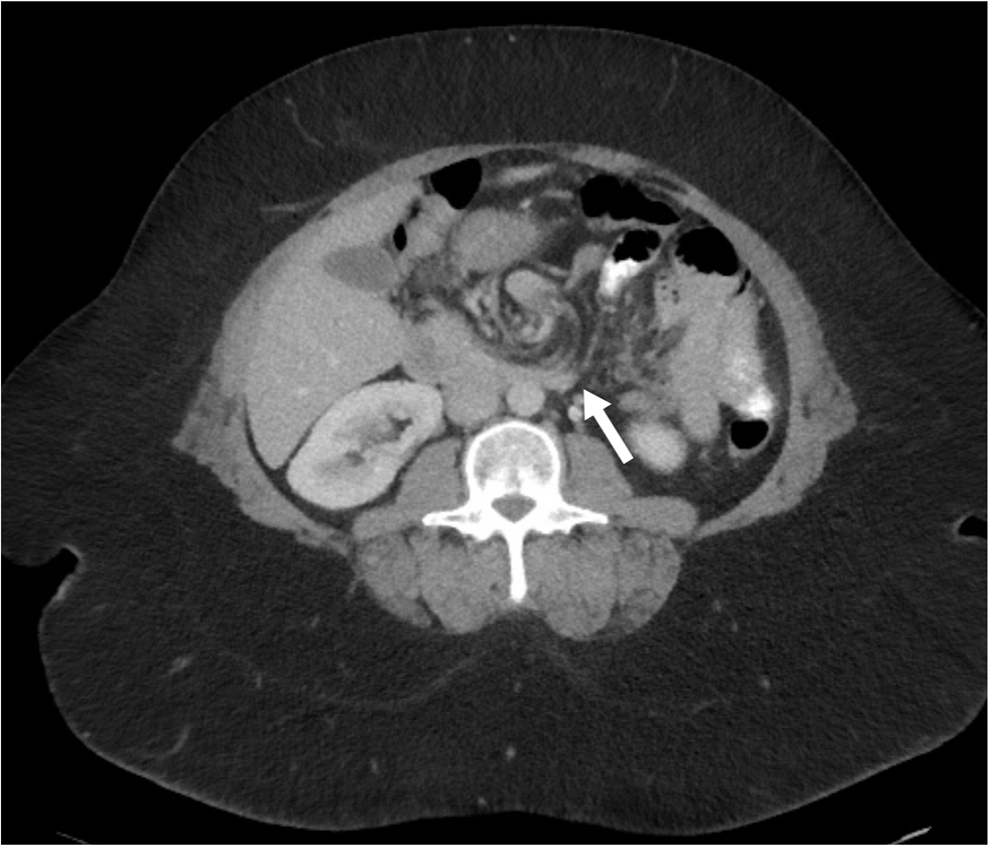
Transverse CT scan through mesentery shows a subtle swirl sign of the mesenteric vessels of a 45-year-old woman with internal herniation
